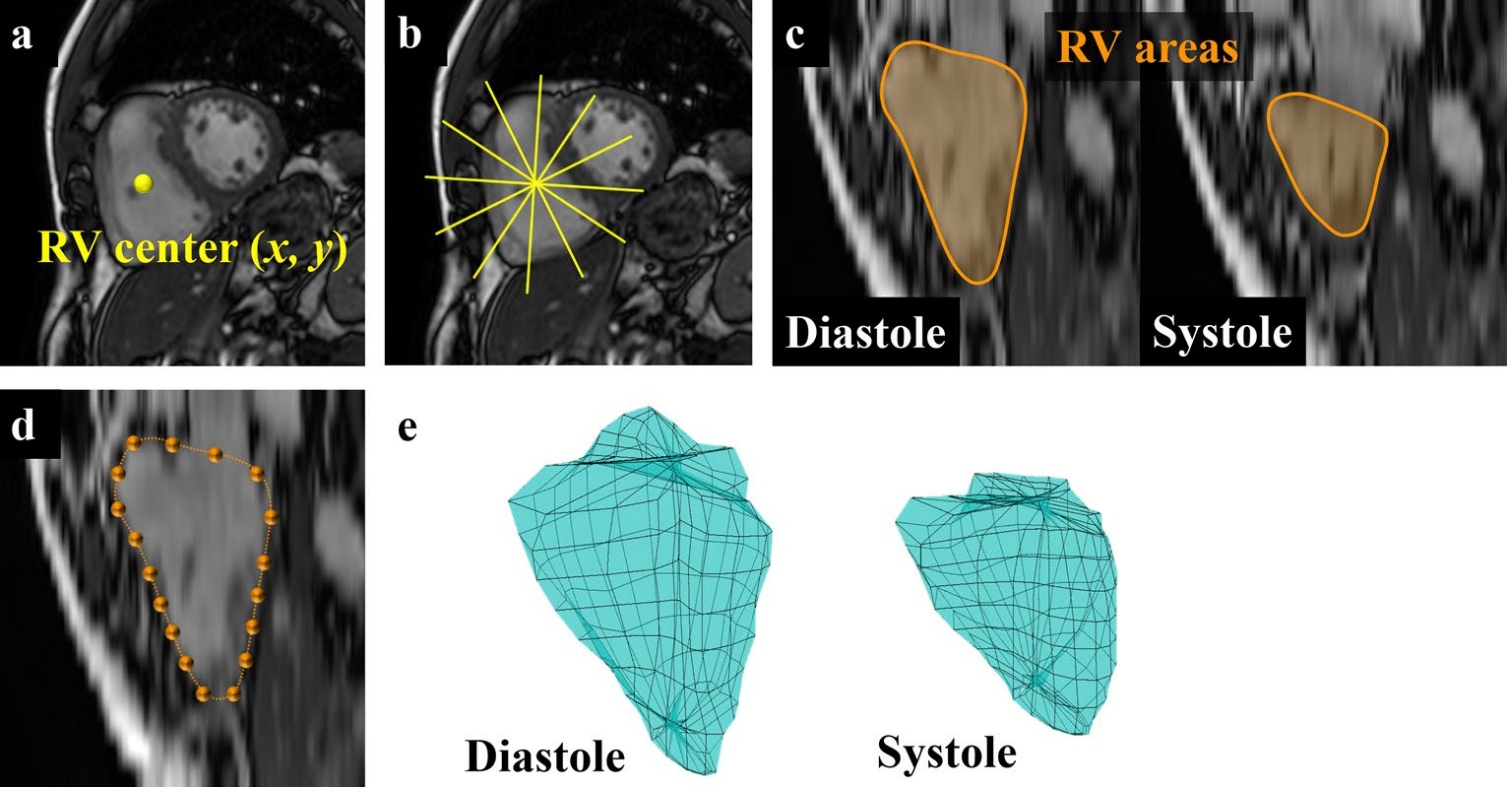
Segmentation of the right ventricle with radial long-axis reconstruction of short-axis cine magnetic resonance images. (a) The point () of right ventricular center is manually determined on the middle slice of the short-axis image in systole. (b) The images interpolated to the slice direction with the nearest-neighbor method are then automatically reconstructed into 6 radial long-axis images (). (c) Right ventricular areas are manually segmented on radially reconstructed images at the end-diastolic and end-systolic frames. (d) Endocardial lines are automatically replaced as evenly distributed points (). (e) Three-dimensional coordinates are automatically obtained at the end-diastolic and end-systolic frames. The right ventricular endocardial surface is individually modeled with these points in each patient.
